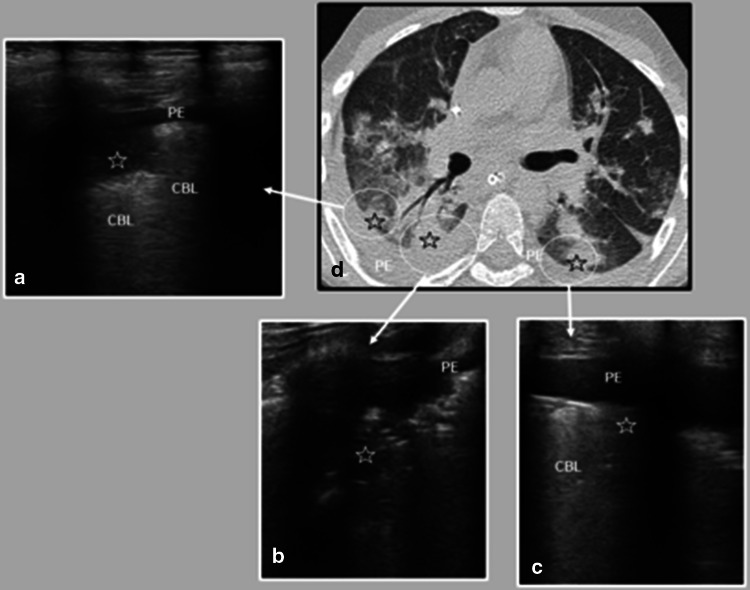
Eleven-year-old male with respiratory failure and shock. Lung US (a–c) was performed on the 5th day after symptom onset and chest CT (d) on the 15th day. There was a pleural effusion (PE in a–d) and subpleural consolidations (stars in a–d). Confluent B-lines (CBL in a and c) were also present in the lung US, corresponding to the chest CT ground-glass pattern
